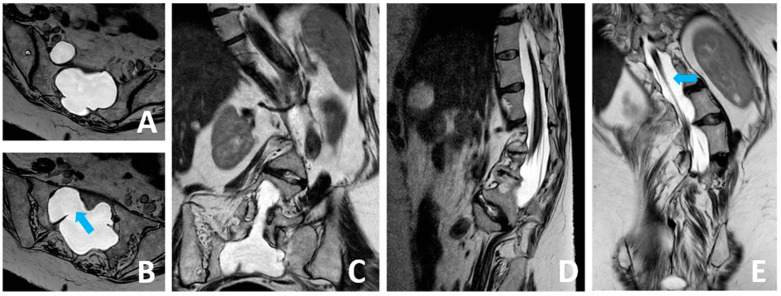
The MRI scan of the whole spine revealed dural ectesia (dAPn 60 mm × dLL 70 mm) in the lumbo-sacral area (A–D). Coronal T2-weighted image showing distal tract of the roots of the cauda equina laterally dislocated (E). The «mega-sac» appeared to be divided and isolated from remaining intrathecal compartment by arachnoid sepiments, as showed in axial T2-weighted images (B), thus resembling a «pseudocystic» dilatation. Blue arrow: arachnoid sepiments in axial (B) and sagittal (E) plane.
